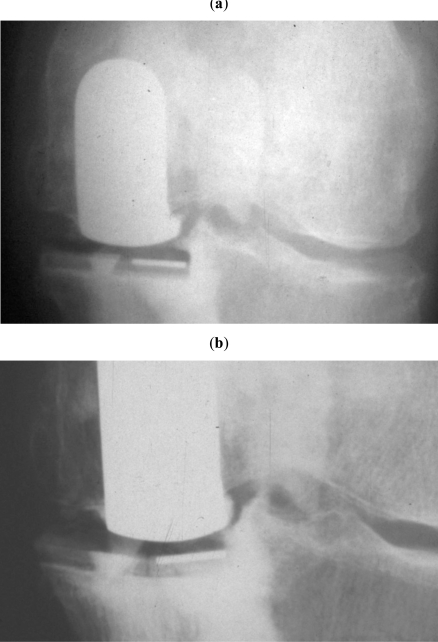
(a) radiograph of an implant 6 mm thick just after the operation. (b) the same implant at a two year follow-up examination = creep is evident on radiograph. Creep of the polyethylene, but also creep of the cement can be observed on the radiograph of the knee with a postoperative varus deformity (hip knee ankle angle: 168 degrees).
